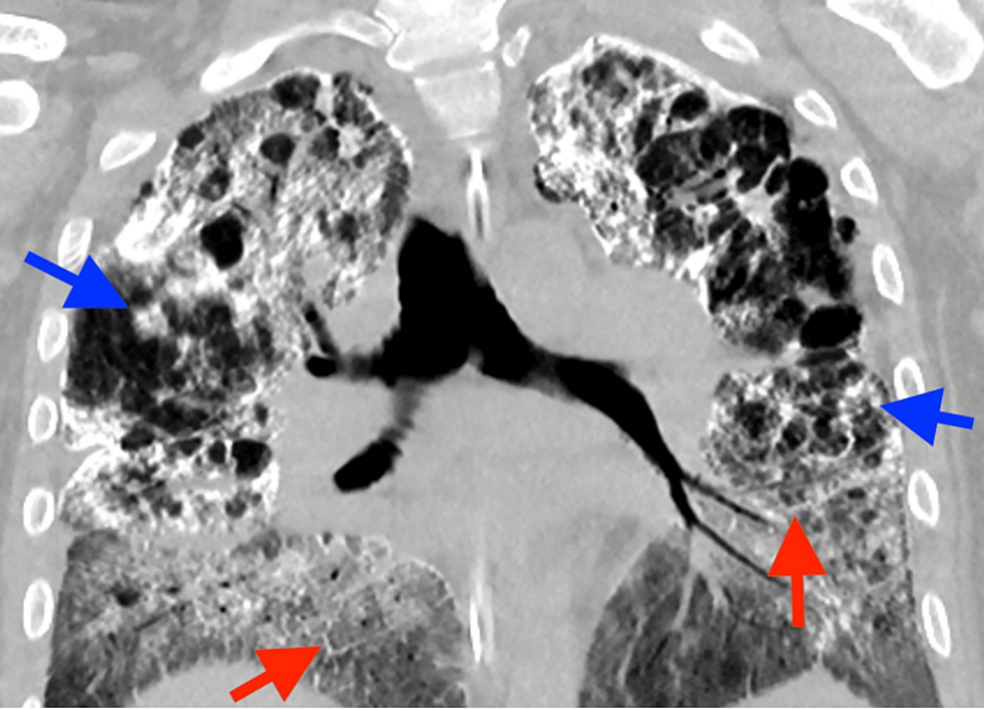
High-resolution coronal chest CT showing diffuse, coalescing calcified micronodules (blue arrows) and interlobular septal thickening (red arrows)
